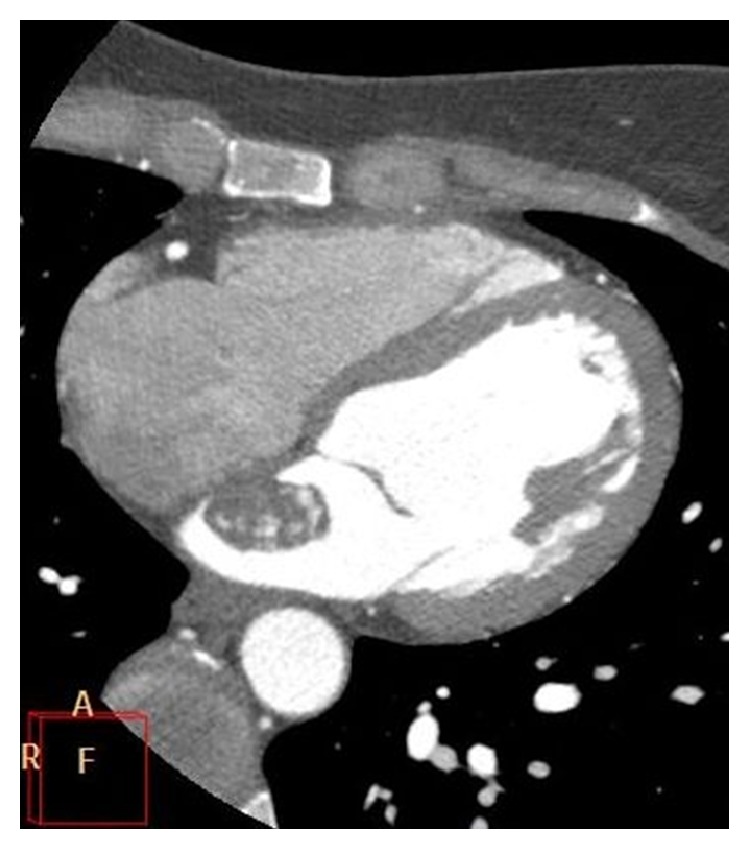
Contrast-enhanced CT image depicting left atrial myxoma attached to the interatrial septum.
